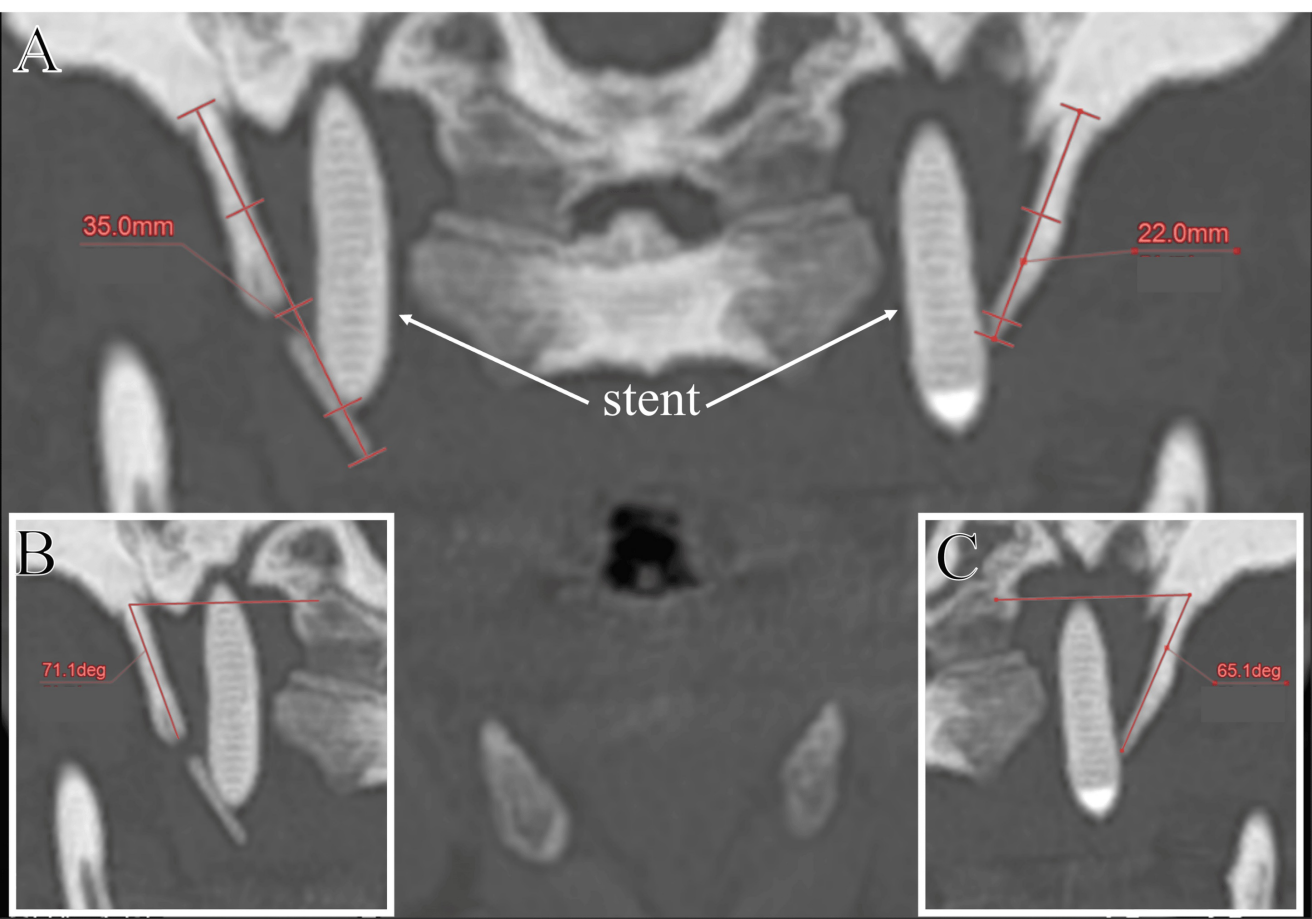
CT scans with maximum intensity projection (MIP) reconstruction before styloidectomies. Coronal plane image showing the SP lengths of 35 mm on the right and 22 mm on the left (A). The medial SP angulation measuring 71.1° on the right and 65.1° on the left, respectively (B-C). The right SP exhibits the morphological characteristics consistent with “calcification of the stylohyoid chain with segmentation”. SP: styloid process
